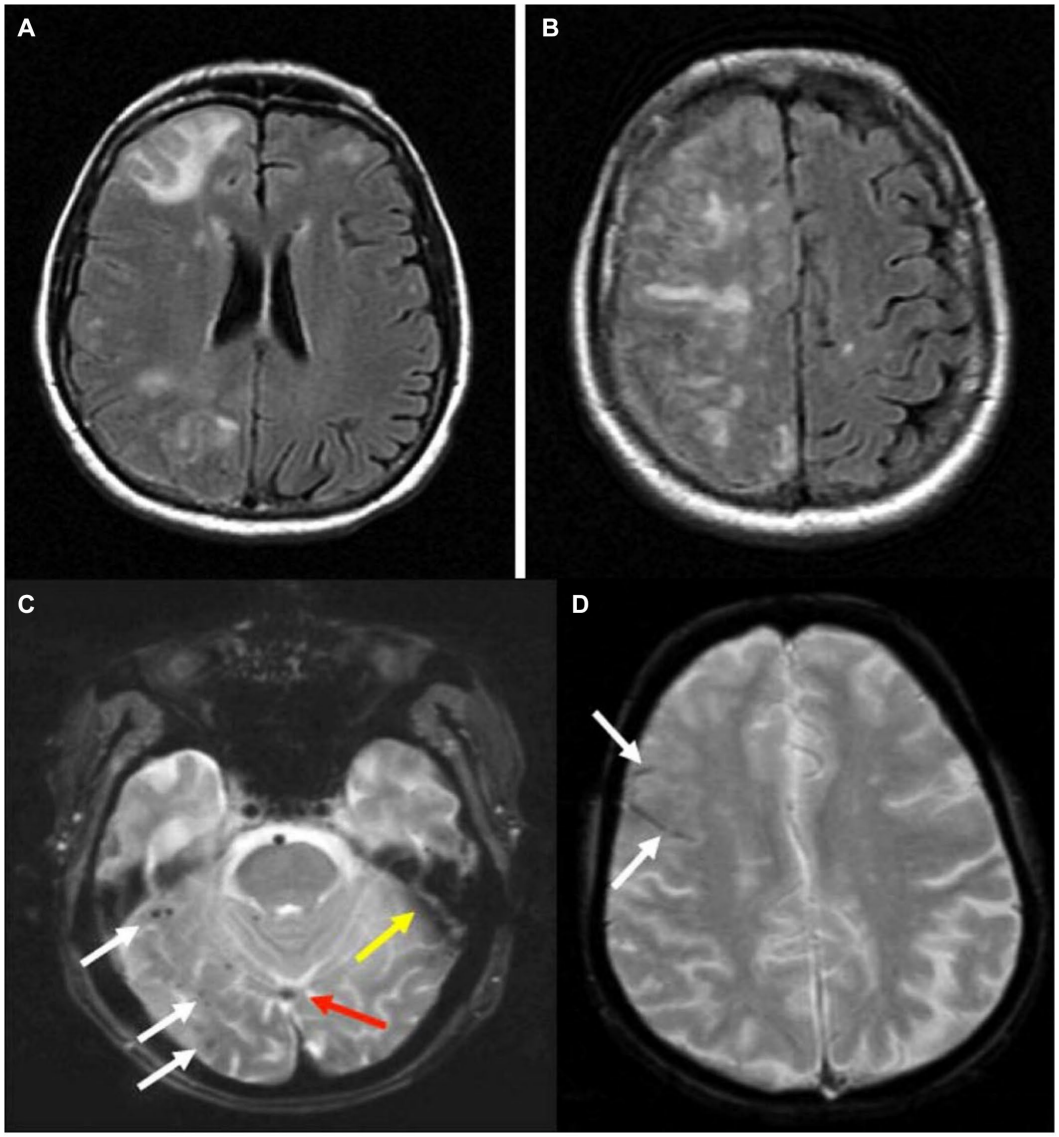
(A,B) ARIA-E which occurred during a monoclonal antibody trial, as seen on fluid attenuation inversion recovery (FLAIR) magnetic resonance images demonstrating increased signal in multiple regions of the right hemisphere affecting both gray and white matter. (C) Microhemorrhage (mH) and superficial siderosis. (C) White arrows indicate multiple 1-to 3-mm dark foci in the right inferior temporal and occipital lobes, typical of the appearance of mH. Red arrow indicates inferior sagittal sinus, and yellow arrow indicates susceptibility artifact, because vascular structures and artifacts can sometimes mimic the appearance of mH and siderosis. (D) White arrows indicate curvilinear dark sulci in the right frontal lobe, typical of the appearance of superficial siderosis. Both images were acquired at 1.5 T with a two-dimensional long TE (30 ms) GRE sequence.
